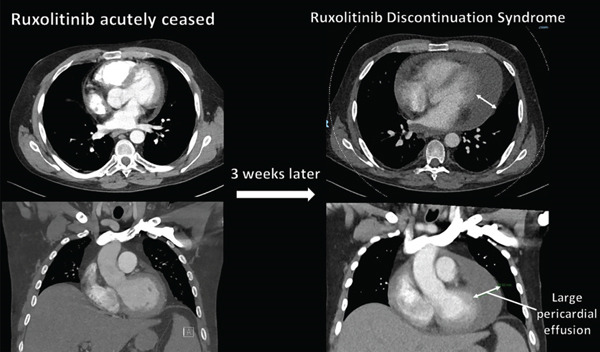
Serial computed tomography pulmonary angiogram (CTPA) showing rapid accumulation of pericardial effusion.
